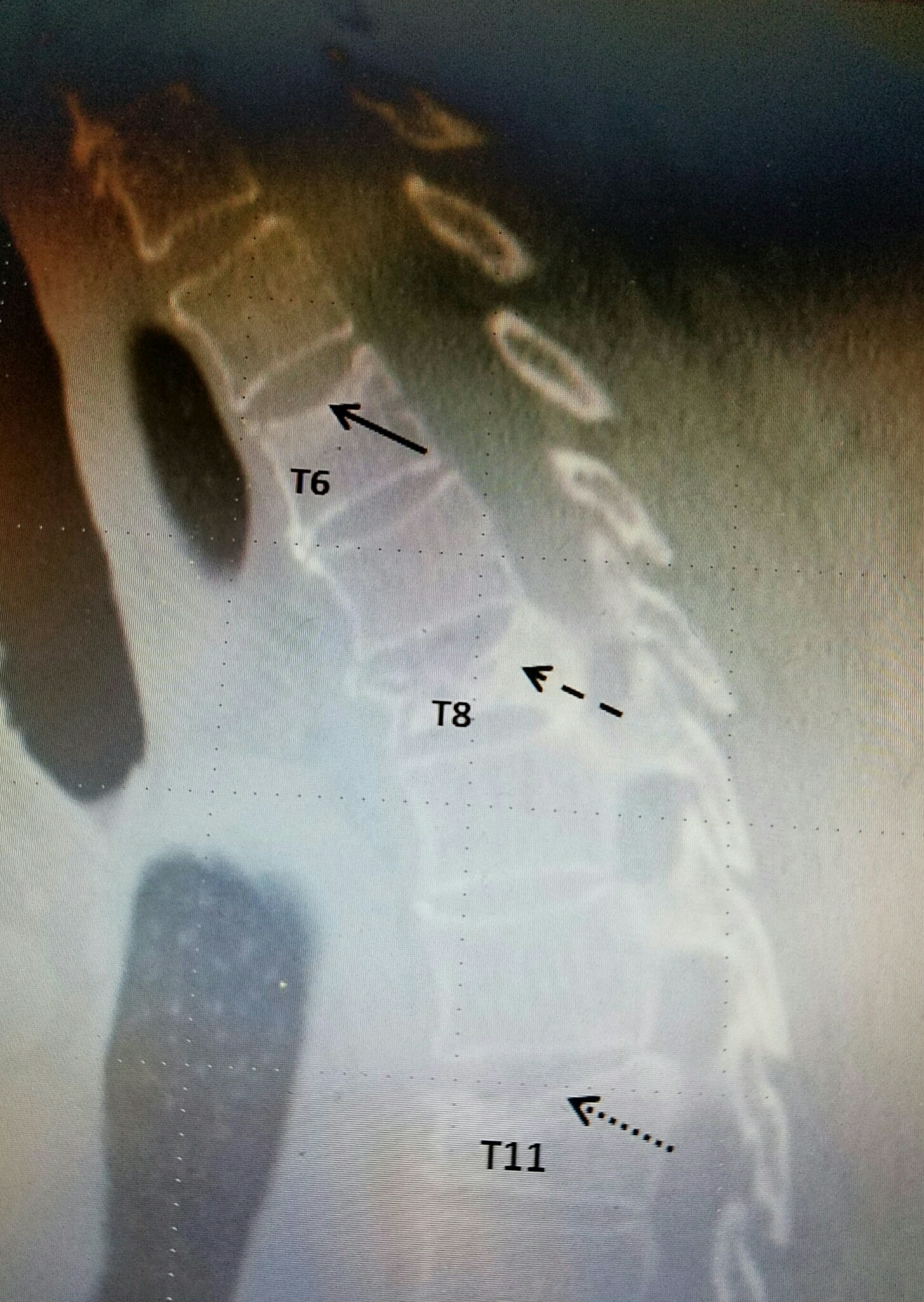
Thoracic spine CT scan sagittal view in a patient who complained of middle to lower back pain. Three vertebral compression fractures are shown at different progressive stages: T6 superior endplate fracture with minimal height loss (solid arrow), T11 compression fracture with moderate height loss (dotted arrow), and T8 compression fracture with severe height loss (dashed arrow)
